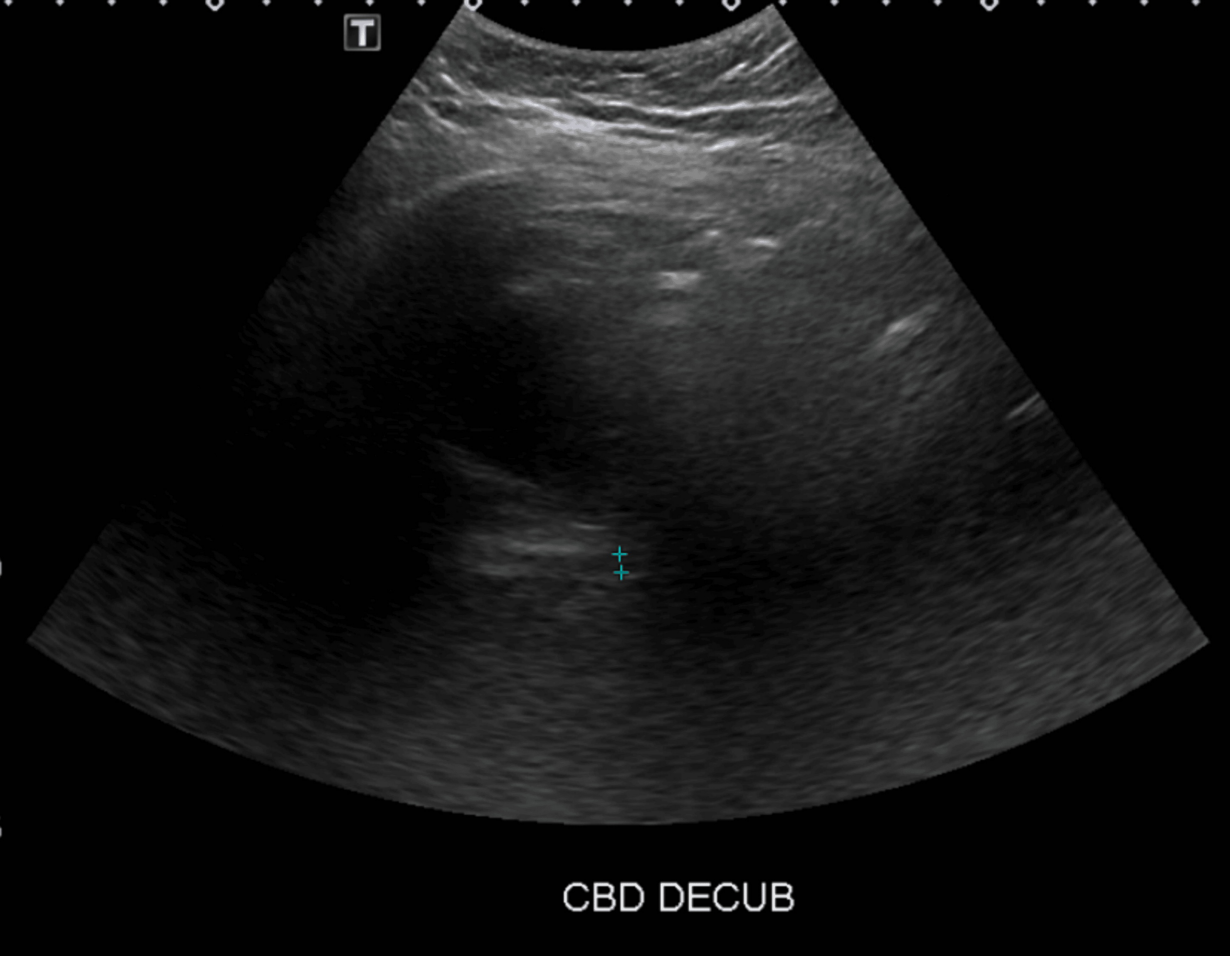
Gallbladder ultrasound in a decubitus position CBD measures 4 mm, which is within normal limits.
